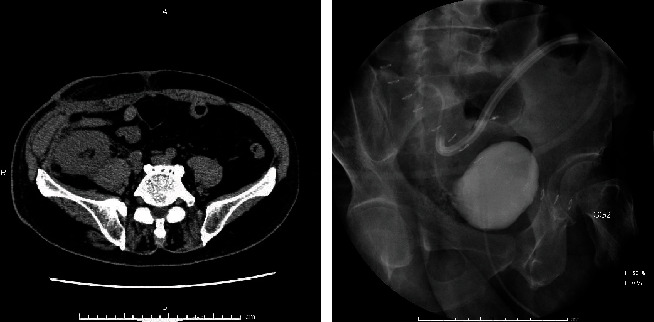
On postoperative day 14, urine leakage is not obvious on cystography. Further, computed tomography revealed no signs of leakage after removal of the drainage tube.
